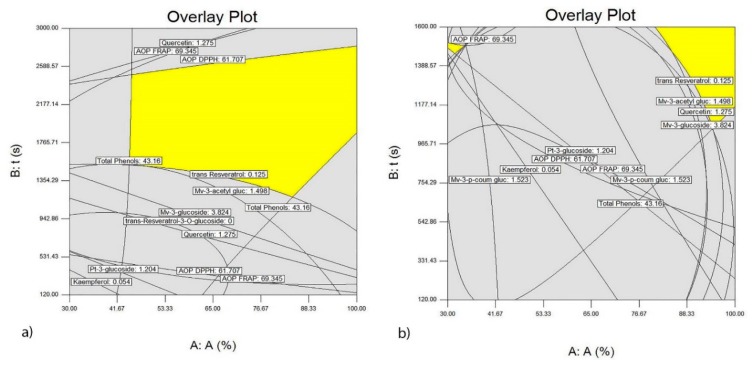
Optimal conditions of the ultrasound-assisted extraction of polyphenolic compounds from the wine lees of the Vranac variety which most significantly affect the AOP of the extracts using the ultrasonic probe with a diameter of 22 mm (a) and using the ultrasonic probe with a diameter of 40 mm (b).
